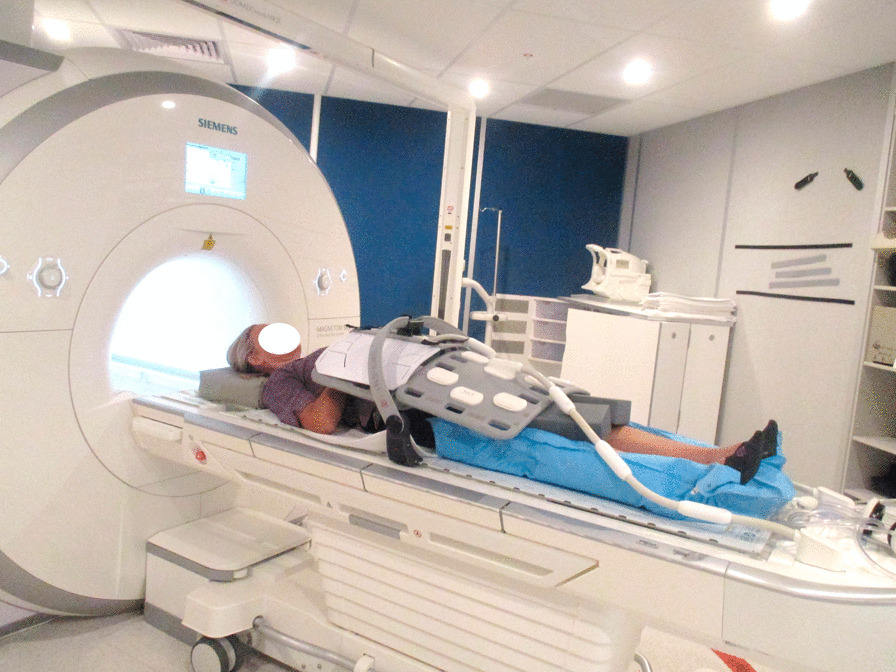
Patient positioning for pelvic MRI using 2 × 18 channel body coils with MRI coil bridge and sponges
